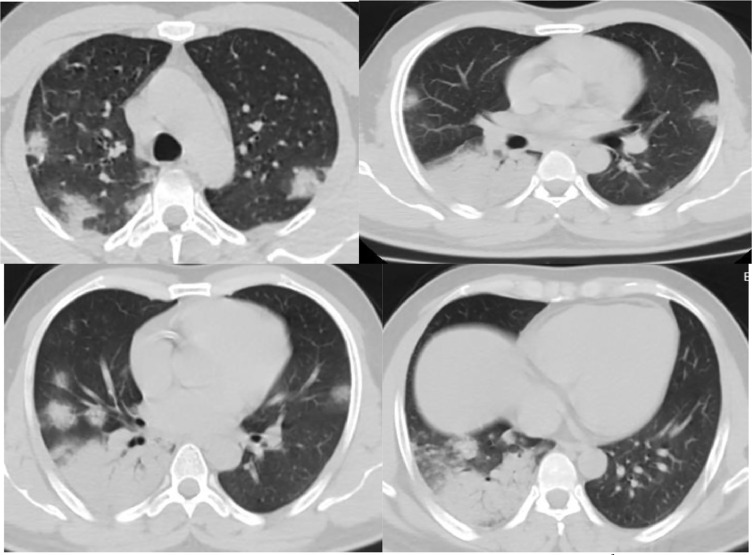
Chest CT scan on 1st day of hospitalization, 3rd day of illness.
